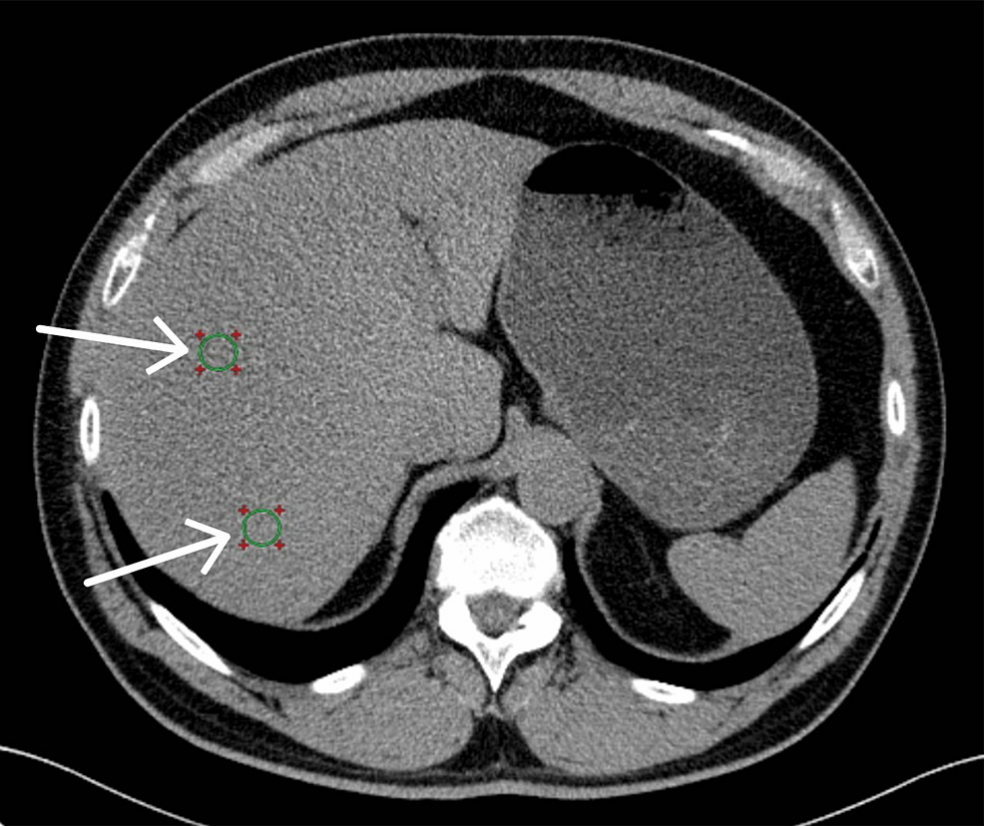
Example image of an axial non-contrast CT slice with the region of interest (ROI) placed within liver parenchyma (white arrows) CT: computed tomography
